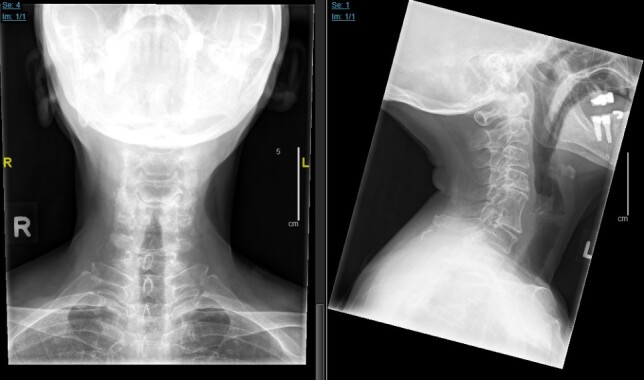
Plain radiographs of the cervical spine demonstrating good bony fusion of C5–C6 and adjacent level degeneration from C3–C7.
